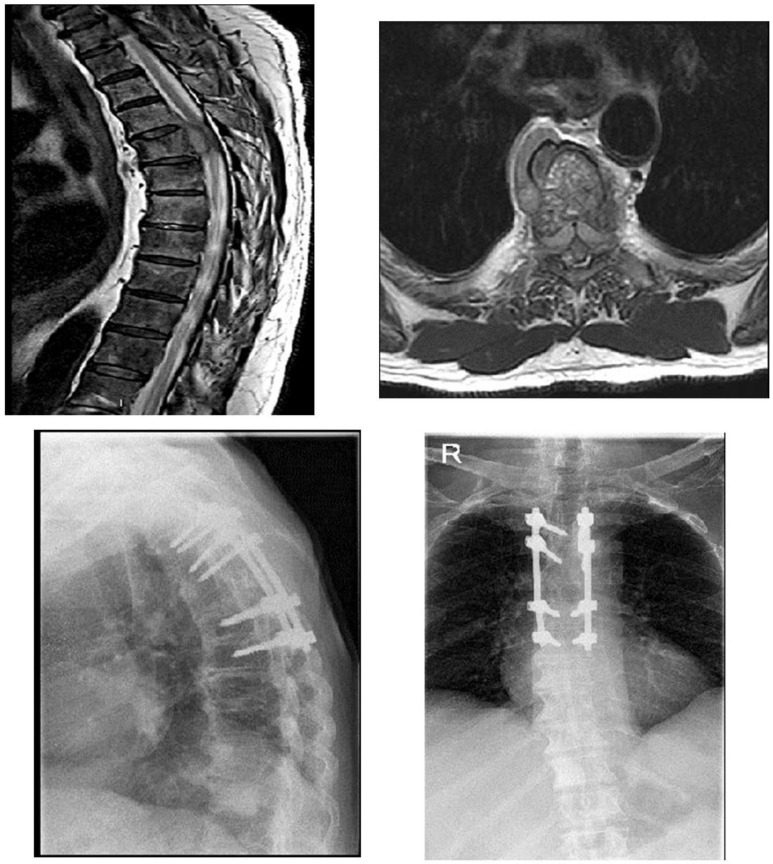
A 78-year-old patient presented to the ER with variable weakness to lower limbs and loss of ability to ambulate. MRI studies revealed a compressing metastasis in D5 as shown on the sagittal and axial T2 images. The patient underwent urgent surgical decompression with partial corpectomy of D5 and stabilization with pedicular screws for two levels above and two levels below the involved lesion. The biopsy revealed a prostate source. Full neurological recovery of motor function was achieved following the surgery.
